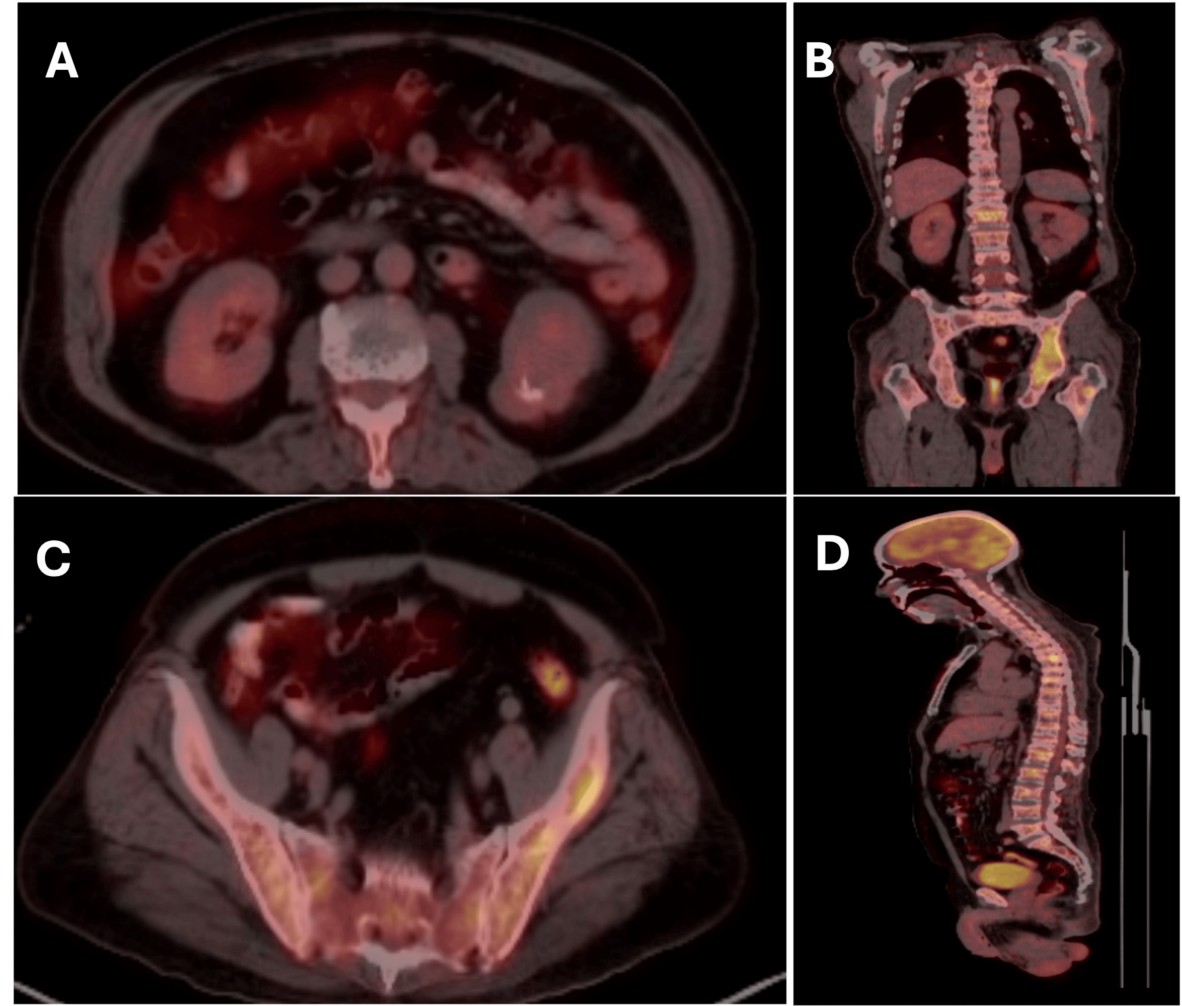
FDG-PET/CT scan displaying low-grade metabolic activity of the renal mass and widespread hypermetabolic lytic skeletal lesions. (A) Fused axial images show low-grade metabolic activity associated with the left lower pole renal mass containing calcifications (SUVmax ≈ 2.7). (B) Fused coronal images reveal extensive hypermetabolic lytic osseous lesions involving multiple vertebral bodies and the pelvis. (C) Fused axial images demonstrate multiple hypermetabolic lytic lesions within the pelvic bones (SUVmax ≈ 8.4). (D) Fused sagittal images show diffuse hypermetabolic lytic osseous lesions affecting the vertebral bodies and posterior elements of the thoracic and lumbar spine (SUVmax ≈ 7). FDG-PET/CT: fluorodeoxyglucose-positron emission tomography/computed tomography; SUVmax: maximum standardized uptake value
